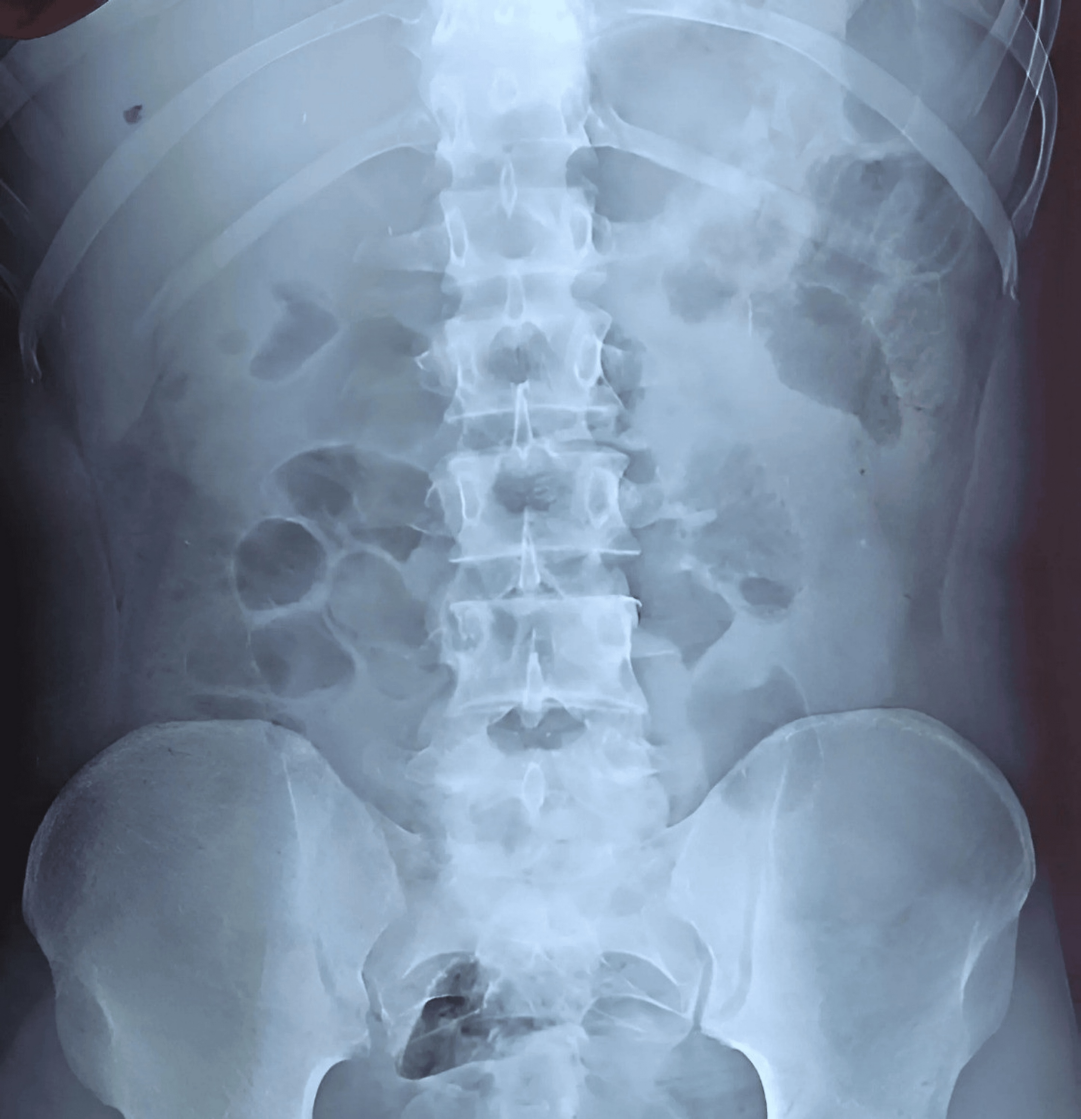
X-ray of the abdomen showing dilated small bowel loops.
